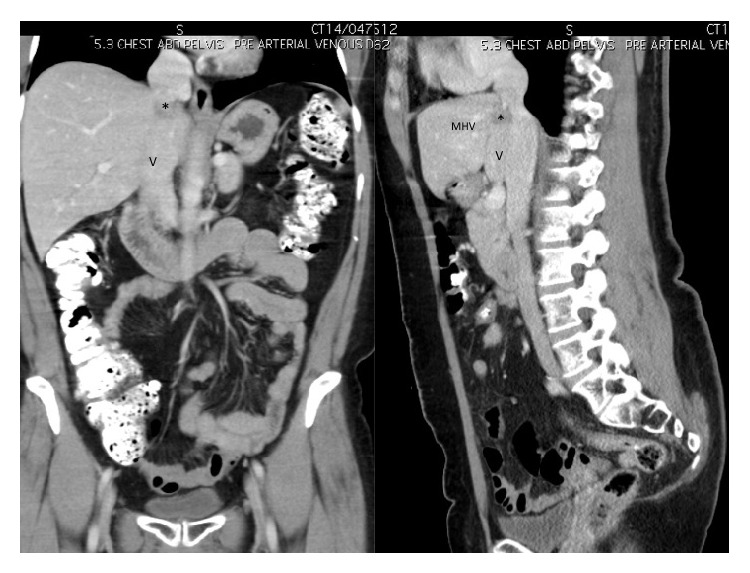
Coronal and sagittal reconstructed CT images showing the IVC (V) receiving the MHV. The solitary metastatic deposit (∗) is seen in segment IVa at the junction of the hepatic-caval confluence.
